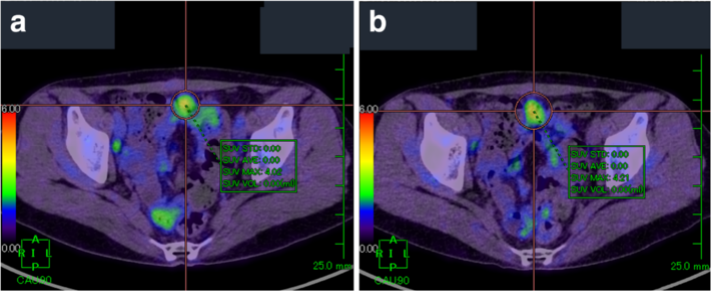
Axial slice on PET-CT after CHOP therapy in the early (a) and delayed phase (b). The lesion had increased in size compared with pre-treatment and showed FDG uptake (SUVmax: 4.02 to 4.21)
